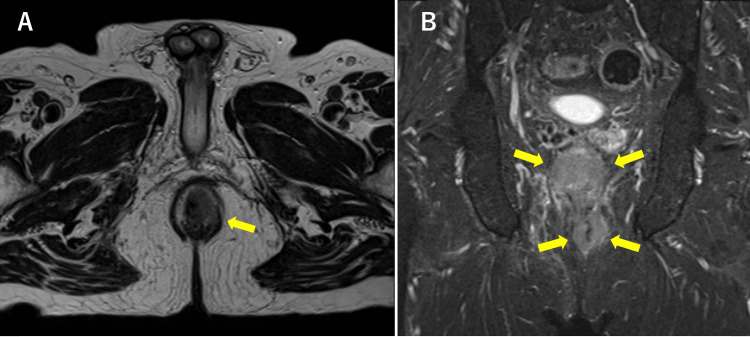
MRI axial and coronal views of Case 2 (A) Axial T2-weighted image showing a tumor lesion in the anal canal (yellow arrow), extending from the 12 o’clock to the 4 o’clock position and invading the perianal fatty tissue. (B) Coronal STIR image demonstrating abnormal signal intensity throughout the entire circumference of the lower rectum. The yellow arrows indicate the tumor extent encircling the anal canal and lower rectum. STIR: short tau inversion recovery
